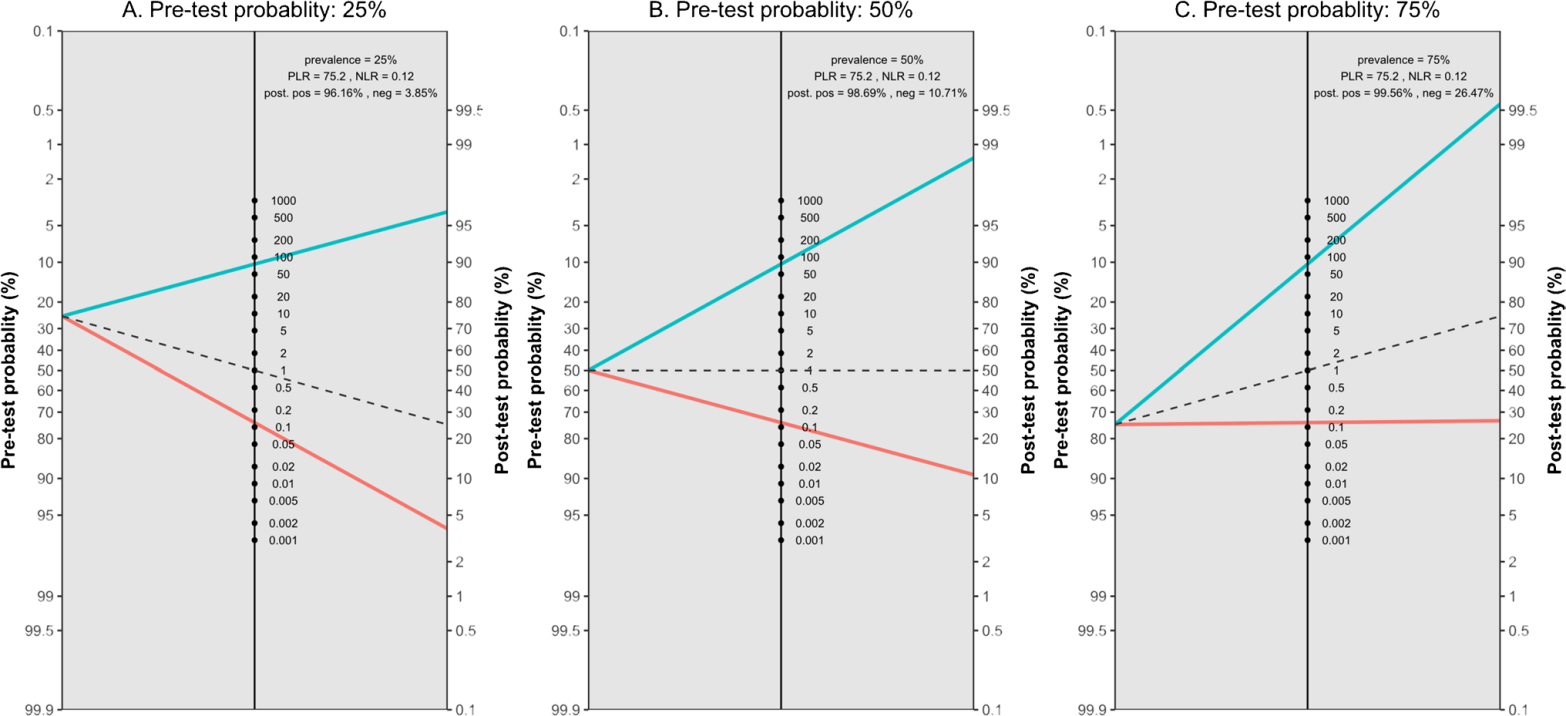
Fagan’s nomogram plots with three different pre-test probability assumptions and pooled diagnostic performance indices based on the bivariate model random effect meta-analysis of diagnostic performance of contrast-enhanced sonography (CEUS) in diagnosing various solid organ injuries in pediatric patients (overall analysis). Neg, negative; NLR, negative likelihood ratio; PLR, positive likelihood ratio; Pos, positive
